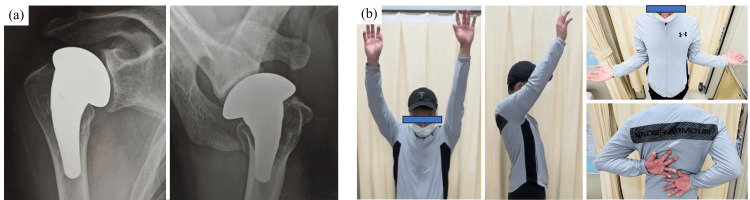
Radiographic findings and shoulder range of motion two years after surgery. Two years after surgery, radiography (a) revealed continued good fixation of the implants, no peri-implant fractures, and fusion of the osteochondral grafts. Active range of motion of the shoulder joint 2 years after surgery (b) was as follows: forward flexion, 120° versus 170°; abduction, 125° versus 170°; external rotation, 40° versus 70°; internal rotation, T10 versus T6.
